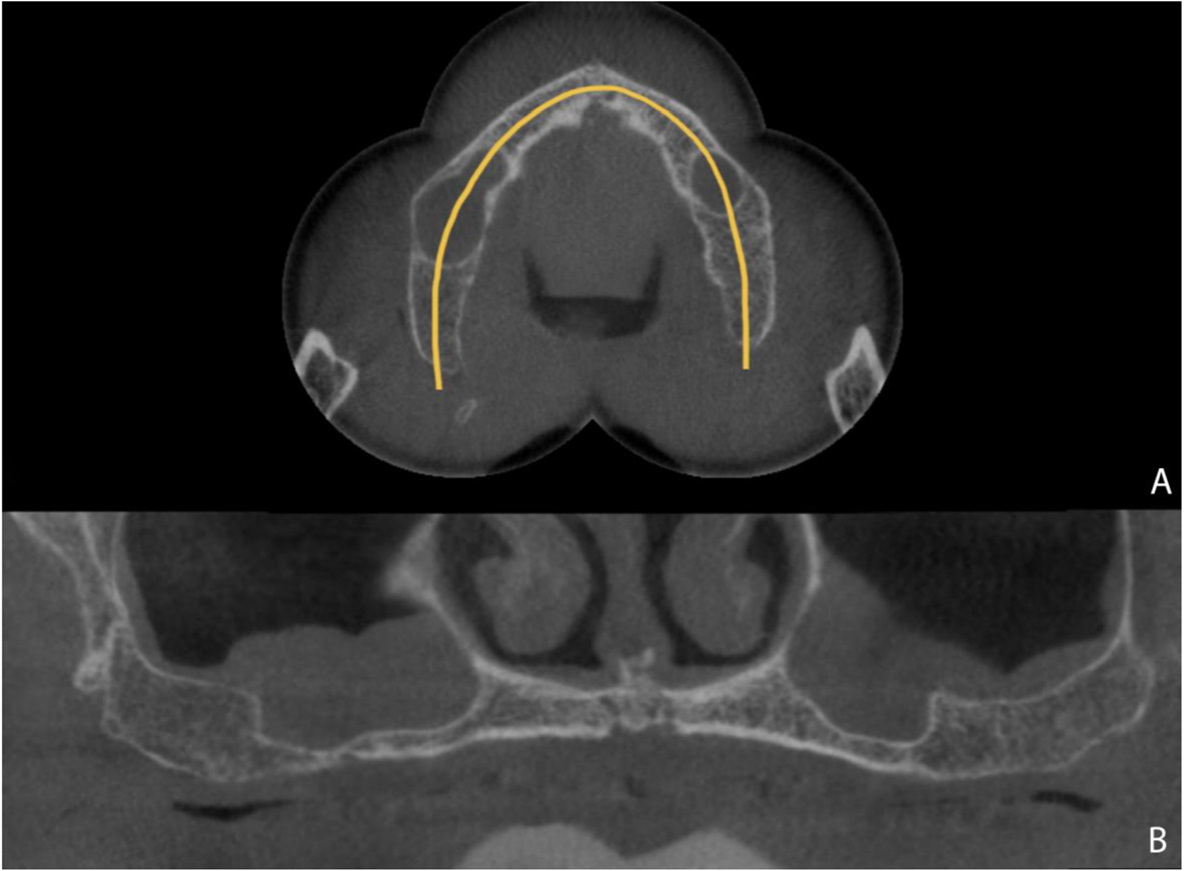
Maxillary computed tomography. a Axial view. b Coronal view
